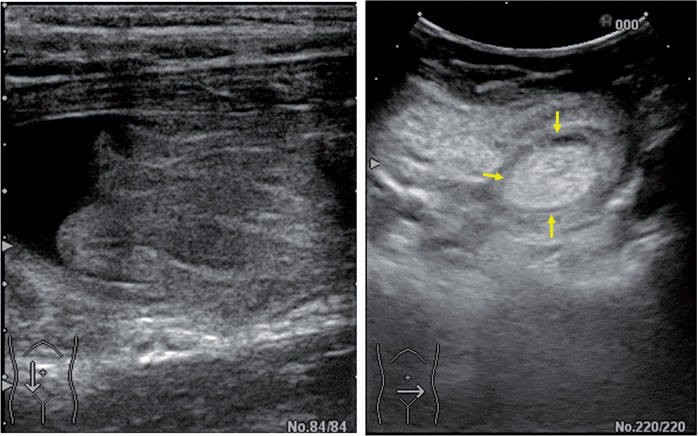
Ultrasonography with transverse view showing echogenic mass, which has pseudokidney appearance.
